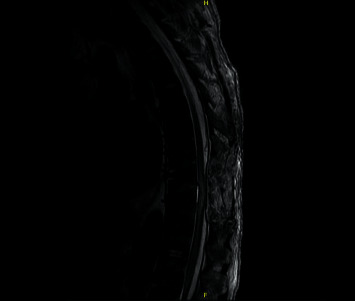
Postoperative MRI sagittal view showing T8–T10 laminectomy with mild dilatation of the cord and a T2 signal abnormality within the cord from the T7-8 through the T10-11 levels.
